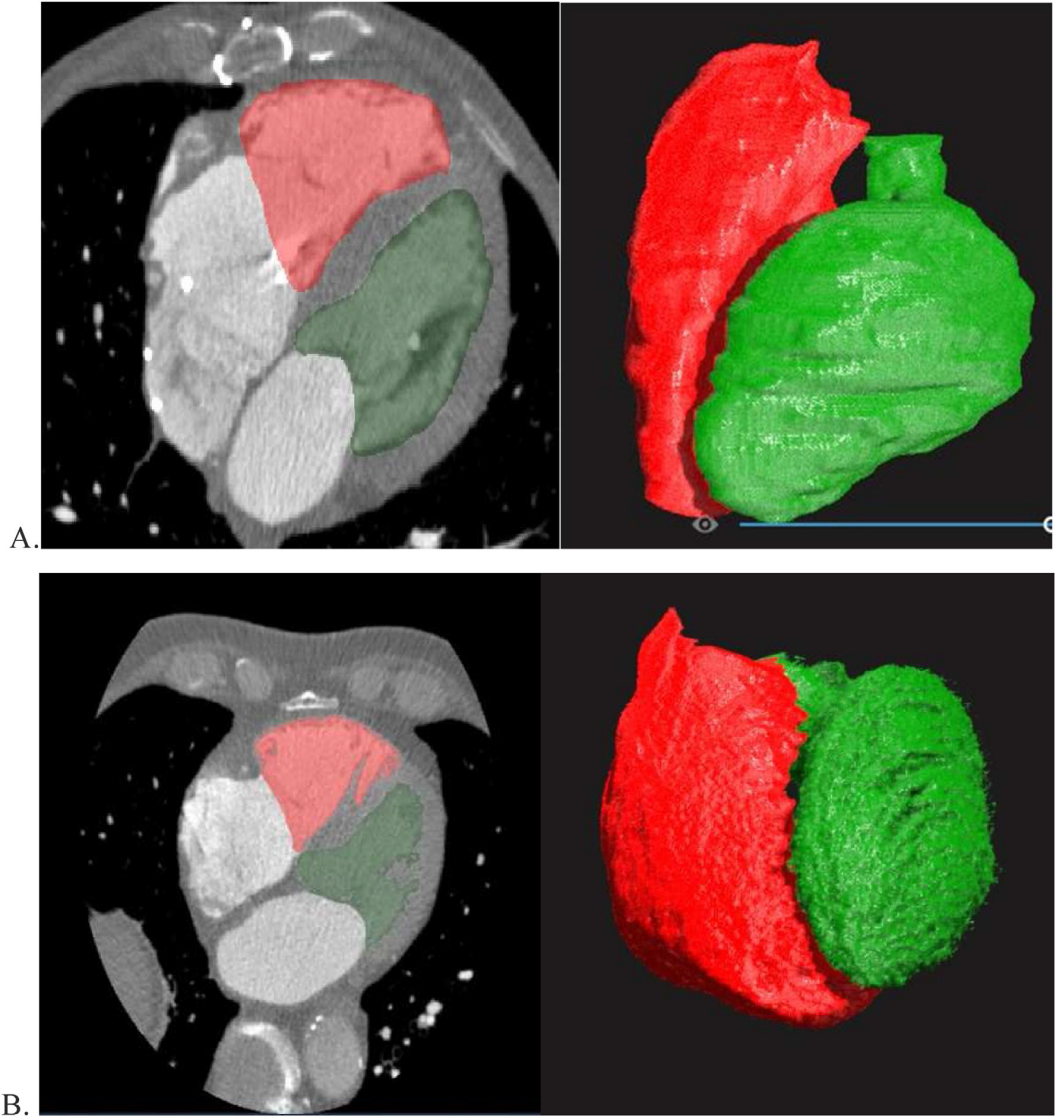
Virtual Reality (VR), three-dimensional imaging, and segmentation including the trabeculae and the papillary muscles produced a smooth border similar to the Philips platform segmentation. A - Cardiac Computed Tomographic Angiography (CCTA) slice and a 3D model with the segmentation border of the left ventricle and the right ventricle, including the trabeculae and the papillary muscles. B - VR imaging and segmentation of the inner blood pool inside the heart chambers.
